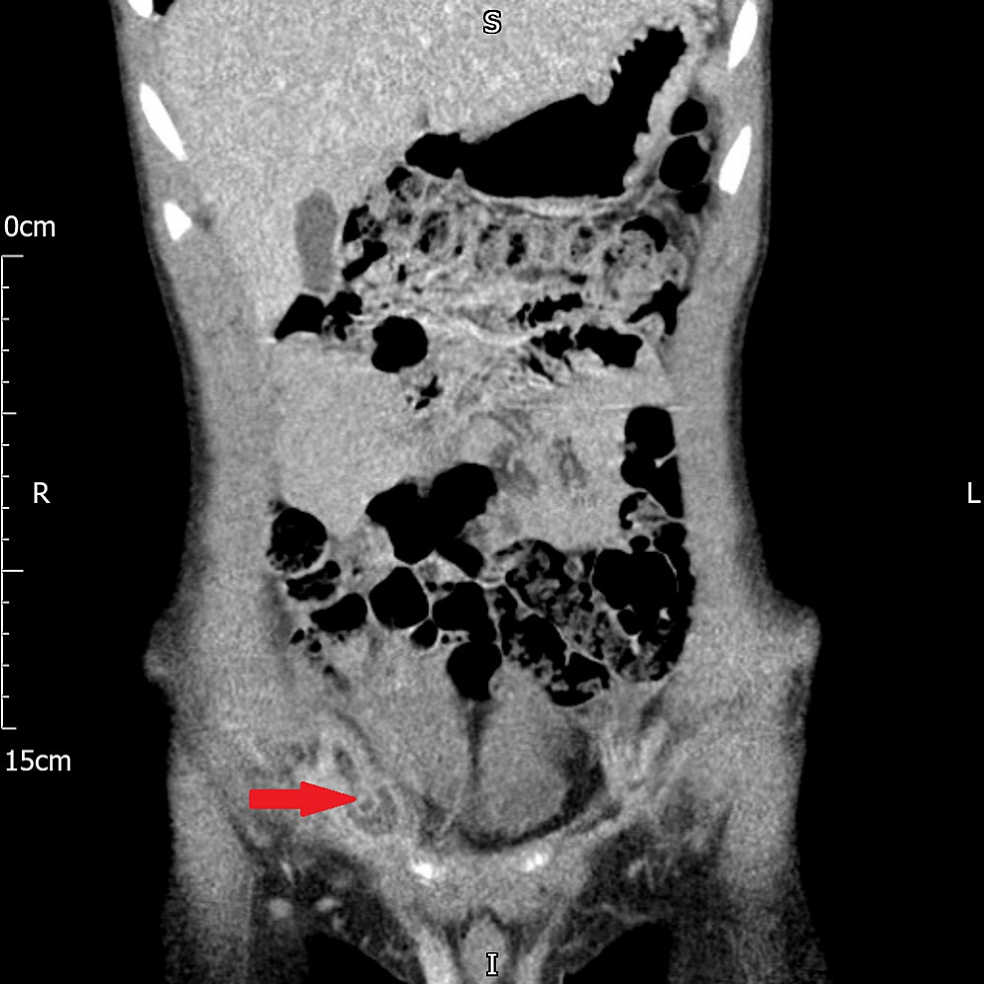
CT coronal view showing the right-sided inguinal hernia, containing the appendix.
